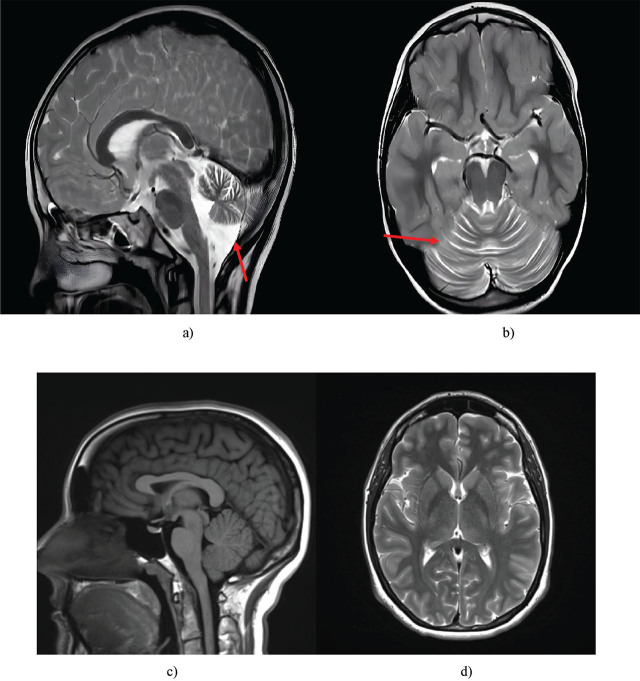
Brain MRI of the youngest child (Patient 3): a) sagittal; b) axial sections*. Healthy brain: c) sagittal; d) axial slices. The pathological features observed include minimal vermian thinning and subtle sulcal widening with relatively preserved cerebellar volume. *Source:* a-b were obtained during the study and c-d are reproduced from [34].
